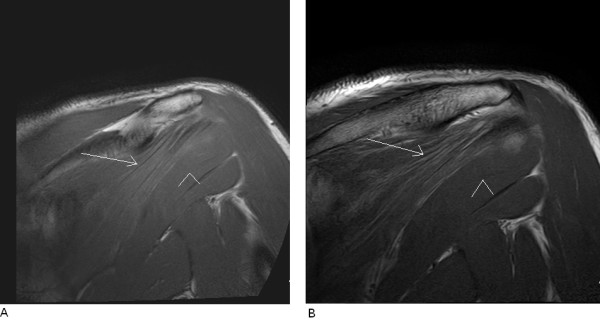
MRI examination performed at 6 months follow up. (A) Oblique coronal T2-weighted/turbo spin echo baseline MRI study of the left shoulder. The long arrow indicates diffuse high signal intensity within the infraspinatus muscle, which suggests fatty infiltration due to denervation. The arrowhead indicates the teres minor muscle, which appears normal in contrast to the infraspinatus muscle. (B) Oblique coronal T2-weighted/turbo spin echo MRI study of the left shoulder obtained during the six-month follow-up examination. The long arrow indicates diffuse high signal intensity within the infraspinatus muscle. The arrowhead indicates the teres minor muscle, which appears normal in contrast to the infraspinatus muscle. These changes are more extensive, and some loss of muscle volume is also shown, suggesting progression of the denervation effects.
